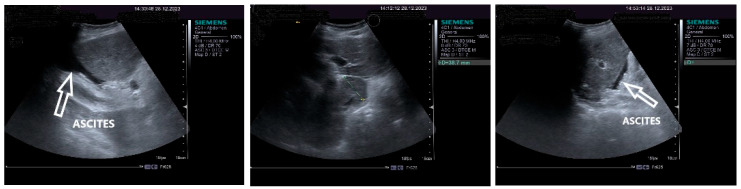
The 2D ultrasound aspect of the liver: perihepatic ascites (right,left), caudate lobe at the upper limit (middle).
